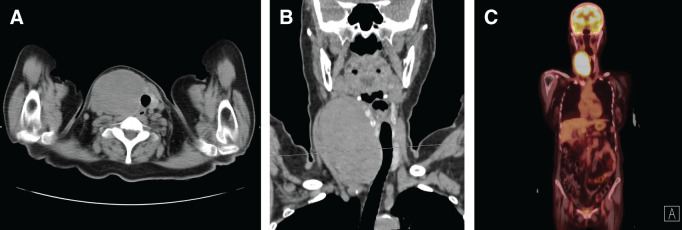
FDG-PET/CT. (A) CT revealed a 93-mm mass within the right thyroid lobe. (B) The mass did not invade the trachea. FDG-PET/CT revealed increased uptake in the mass. (C) No evidence of lymph node involvement or distant metastasis was observed. FDG-PET/CT, 18F-fluorodeoxyglucose PET/CT
